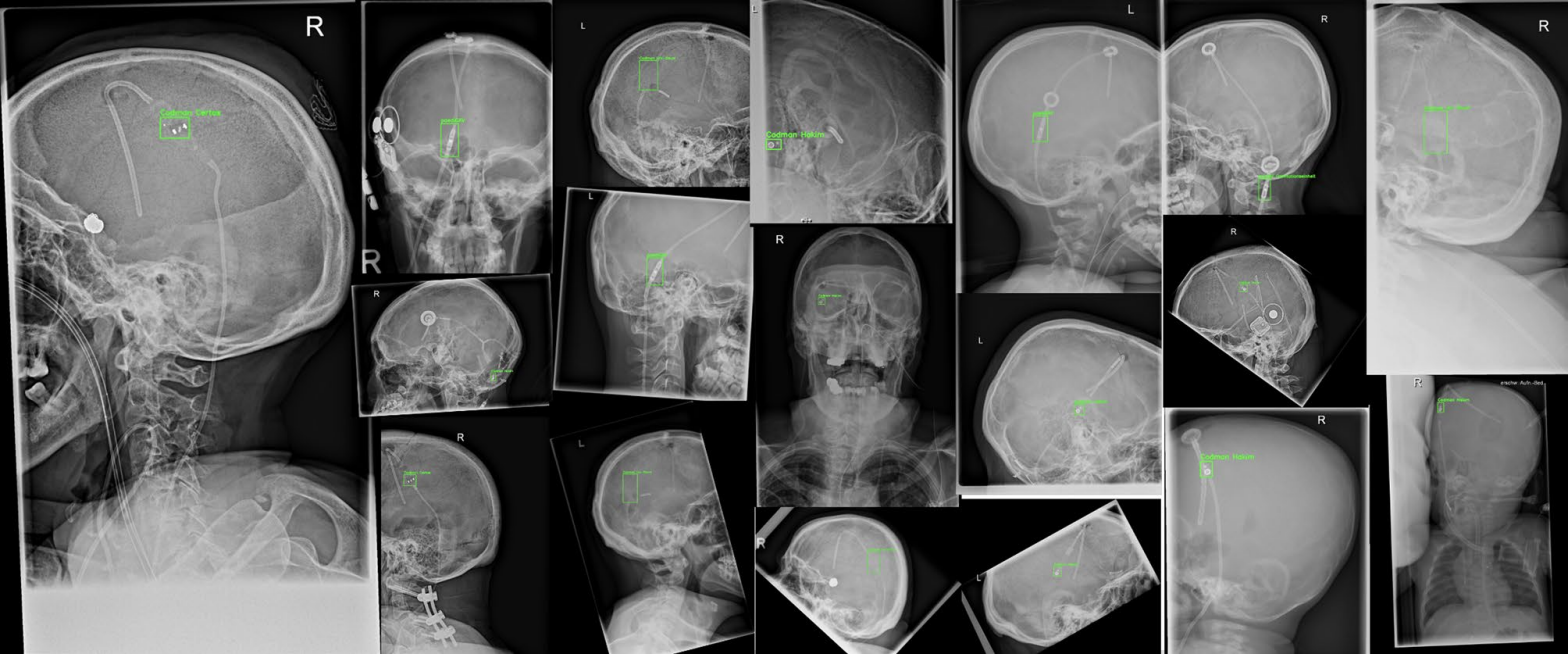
Representative X-ray images from the dataset, each showing a detected CSF shunt valve marked with the predicted bounding box.
